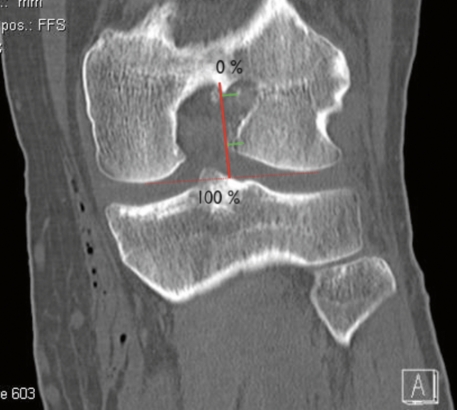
Measurement of the femoral tunnel on the coronal CT reconstruction, performed according to Hoser et al. (2005). The tunnel is measured in comparison to the line perpendicular to the most distal points of the femoral condyles. The measurement is compared to the line from the intracondylar roof to the distal femoral condyles.
